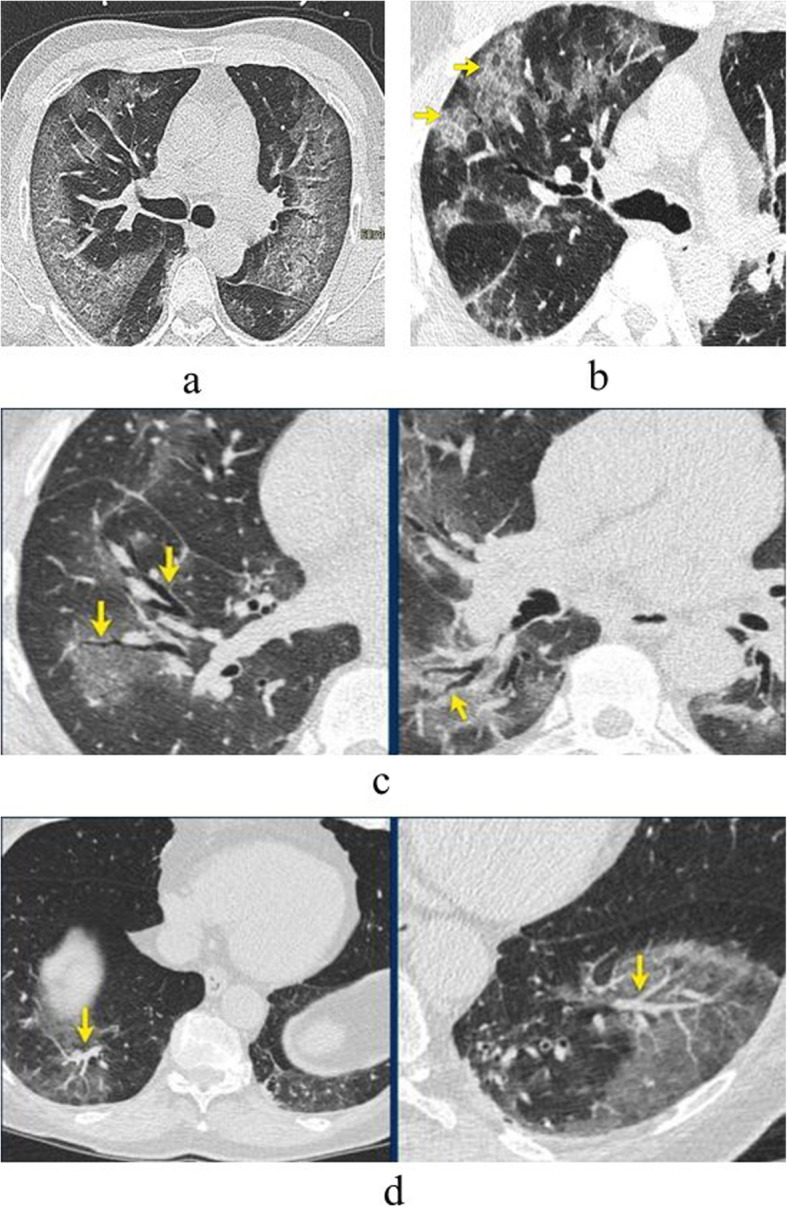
Common image findings observed in lung CT scans of the patients infected with COVID-19. a Ground-glass opacity; b Crazy paving; c Traction bronchiectasis; d Vascular dilation [26]
